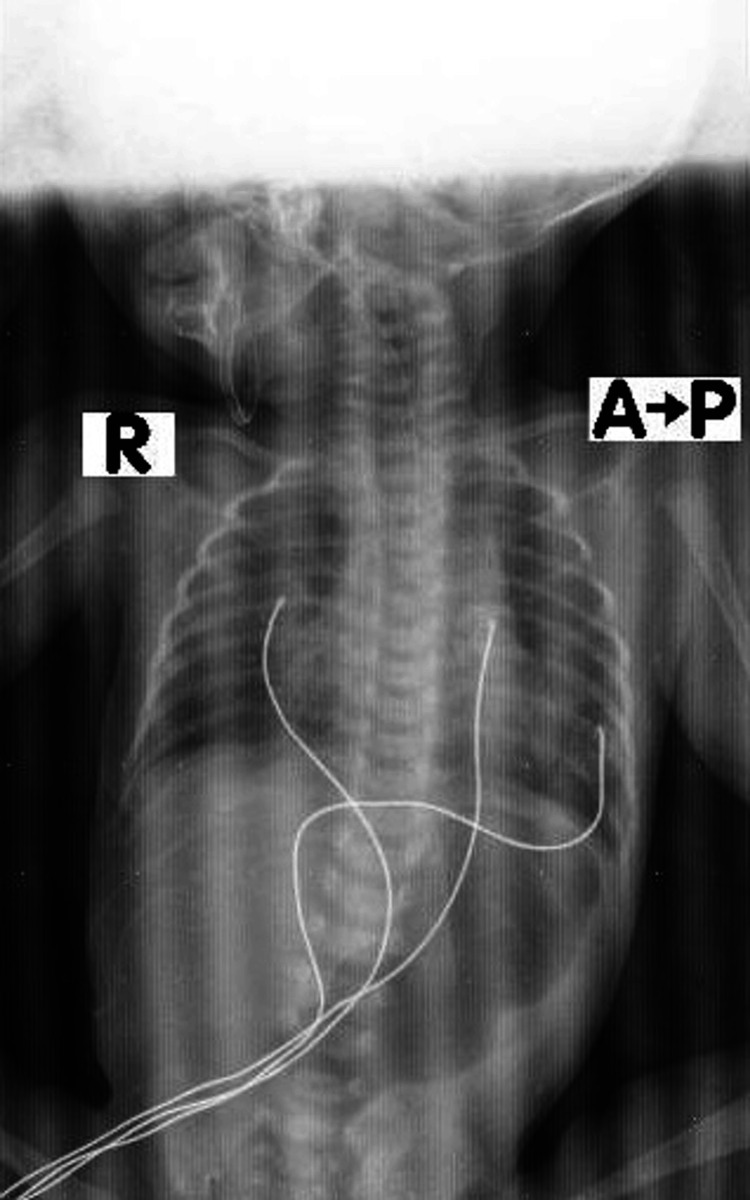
Radiographic representation of DA DA, duodenal atresia
